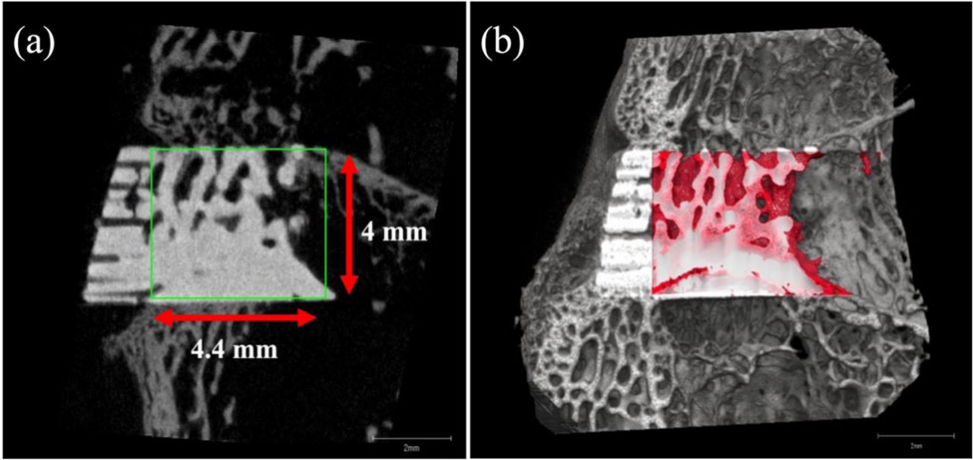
a Region of interest (ROI) was defined as 4.4 and 4 mm in a coronal view. Volume/tissue volume, trabecular bone thickness, trabecular number, and trabecular separation were measured within the ROI. b The red areas in the 3D CT image indicated new trabecular bone formation
